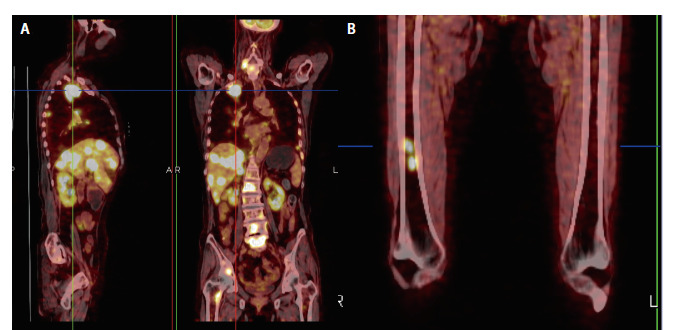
PET/CT扫描结果。A：右肺原发病灶和广泛的转移病灶；B：骨性骨质破坏。
